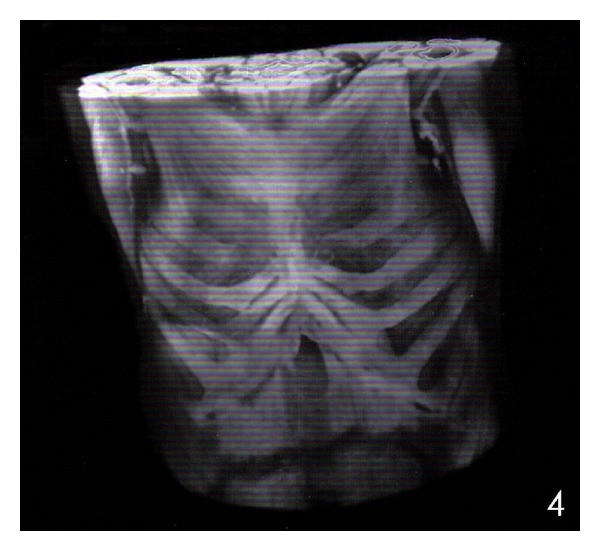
Postoperative chest wall MRI.
